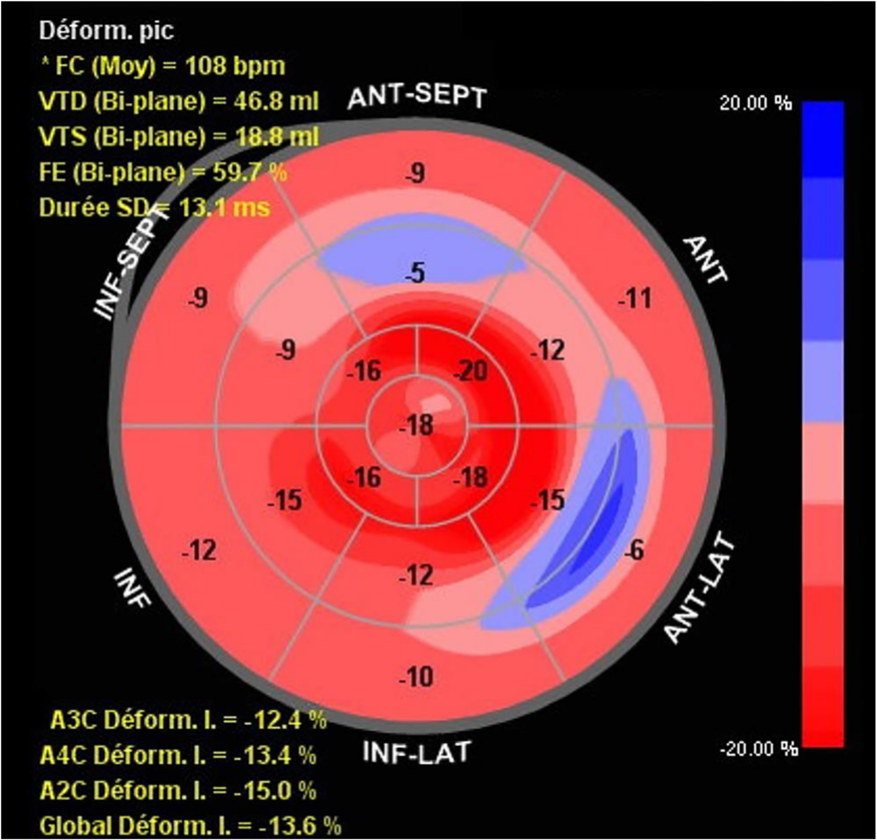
Bull’s eye plot obtained after myocardial strain imaging in a patient with severe SARS-CoV-2 infection. Apical segments are highly contractile (red color) while anteroseptal and anterolateral segments (blue color) are hypokinetic. Calculated strain in apical 4-chamber view was -13.4% and GLS was -13.6%, indicating reduced LV longitudinal function. LVEF was normal (Simpson biplane: 59.7%)
